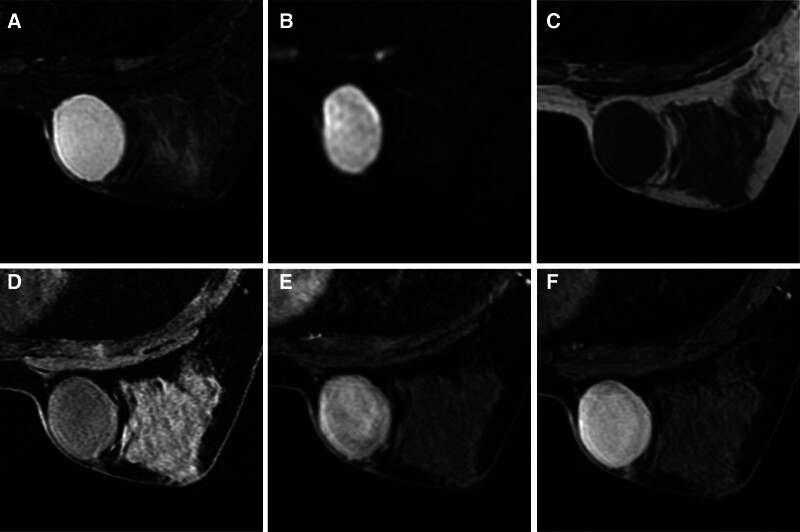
Axial fat-saturated, T2-weighted image (A) and diffusion-weighted image (B) reveal hypointensity oval mass. In axial, nonfat-saturated, T1-weighted images (C) and pre-scan, fat- saturated, T1-weighted images (D), the mass exhibits slightly hypointense when compared to the surrounding breast fibroglandular tissue. After contrast medium administration, the mass shows defined, fast enhancement in the initial phase (E) and persistent in the late phase (F).
